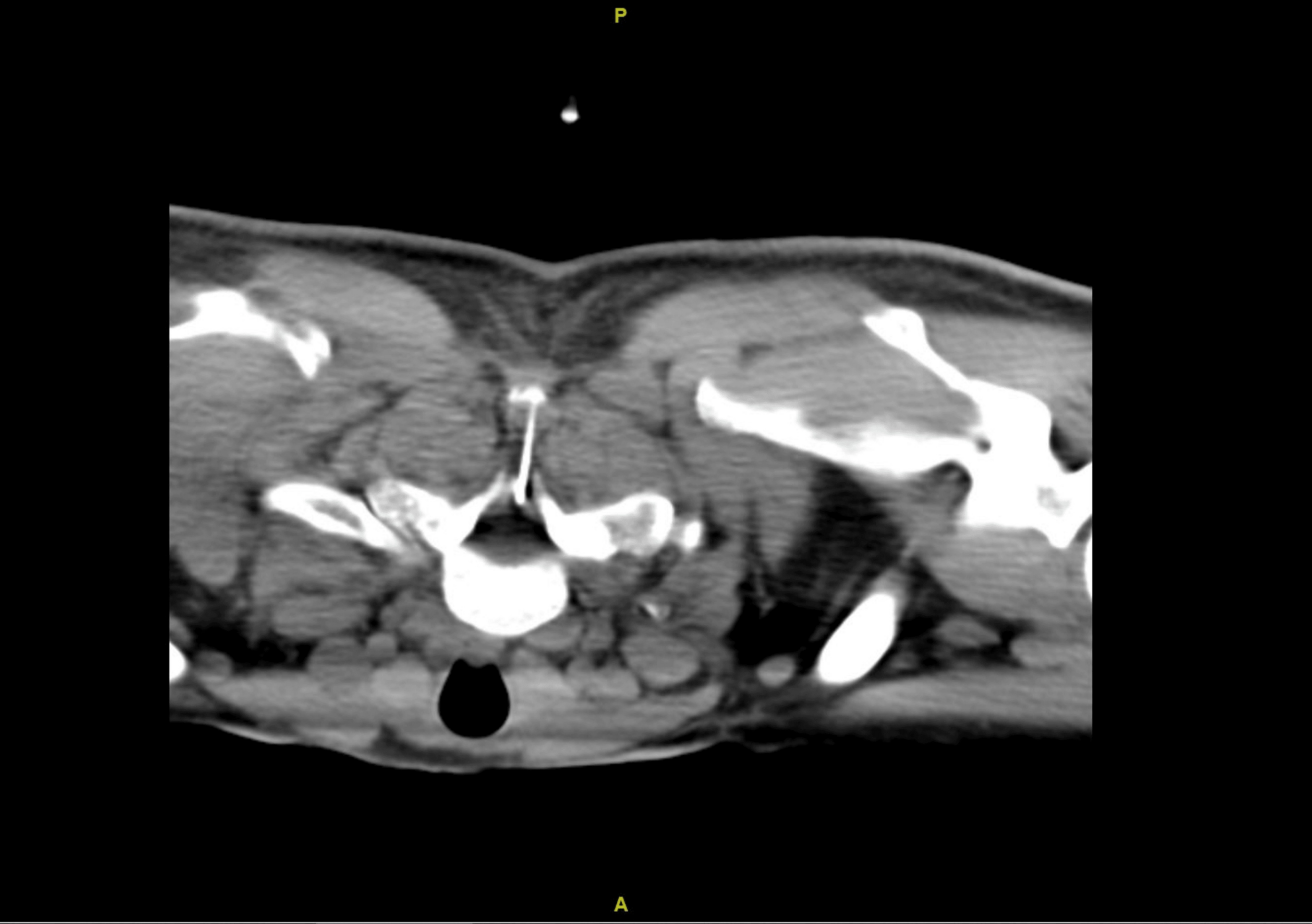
Position of the spinal needle confirmed with CT.
